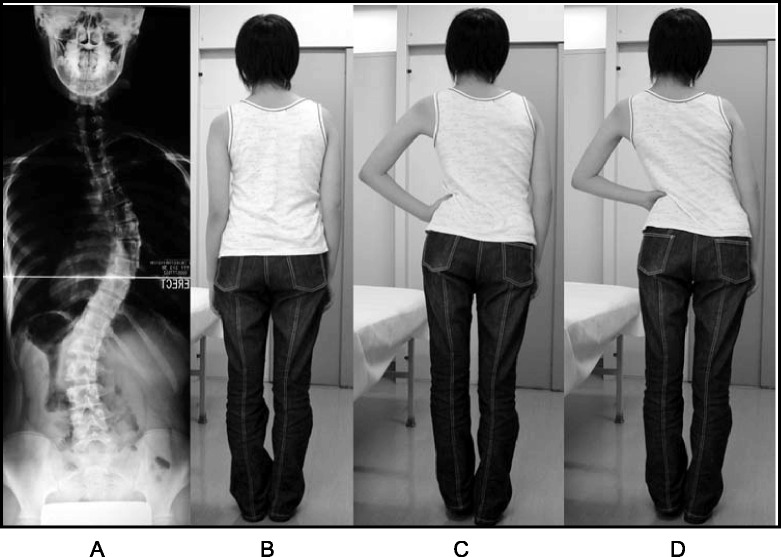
(a, b, c, d): The “Hitch-Shift” exercise is indicated for patients with double scoliosis curves. A patient with a double scoliosis curve seen on radiograph (a) stands in a neutral position (b). She is instructed to transition into the “hitch” position (c) by lifting her left heel on the same side as the convexity of the lumbar curve while keeping her hip and knee straight. She then immobilizes the lumbar curve using her hand and “shifts” her trunk to the concavity of the thoracic curve (d)
